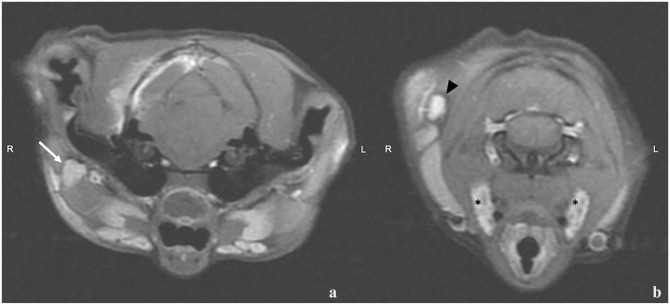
Transverse T1-weighted postcontrast images of the brain at the level of (a) the tympanic bulla and (b) the atlantooccipital junction. Note the moderately enlarged right parotid (arrow), right lateral retropharyngeal (arrowhead) and bilateral medial retropharyngeal (*) lymph nodes
